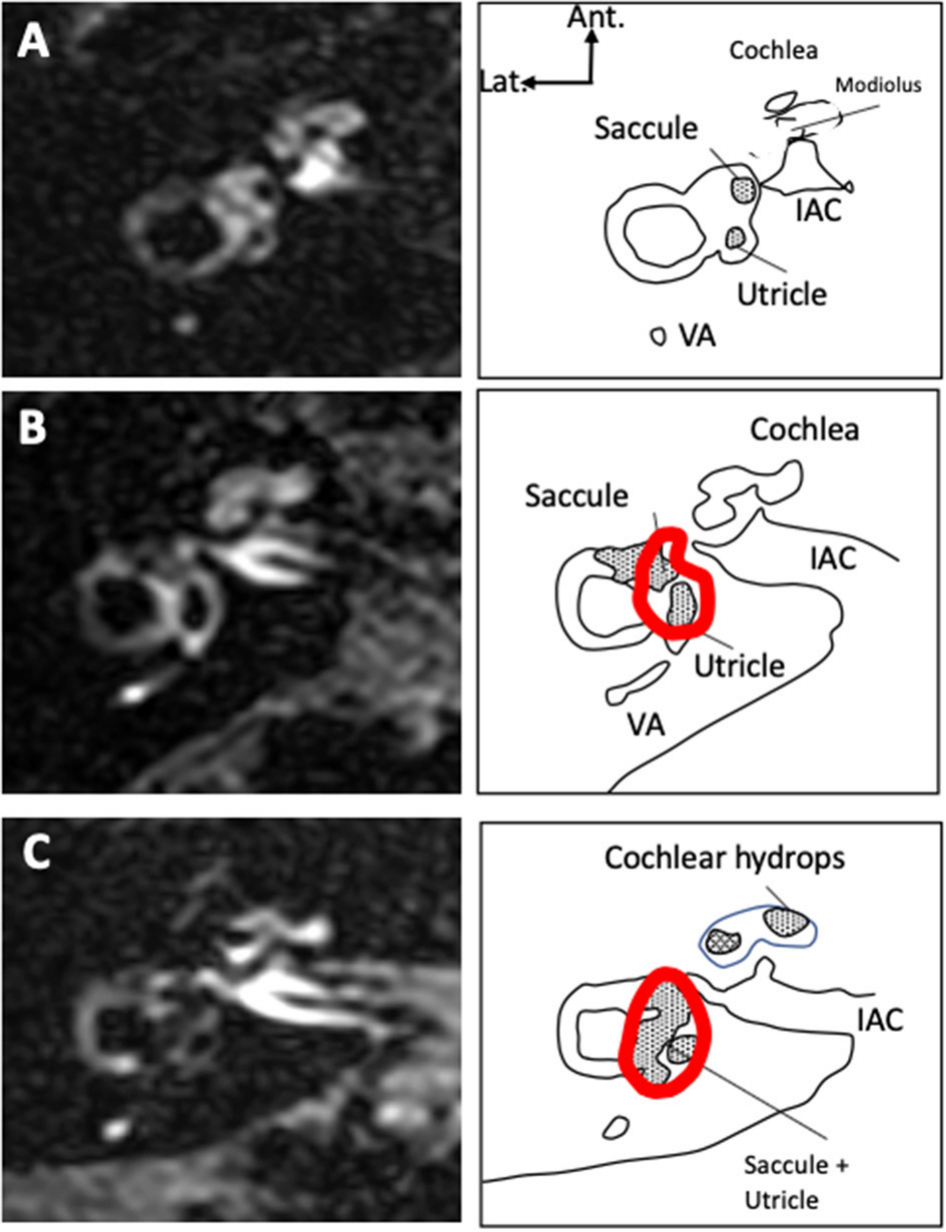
Vestibular and cochlear hydrops on 3D-FLAIR axial views 4 hours after IV gadolinium. Examples of ears with no signs of hydrops [grade 0, (A)], with moderate hydrops [grade I vestibular and cochlear hydrops, (B)], and significant hydrops [grade II vestibular and grade I cochlear hydrops, (C)] are presented. The total surface of the vestibule as measured in the study is contoured in red. Only the saccular surface inside the vestibular area was measured. In case of extensive hydrops (C), the saccule could not be distinguished from the utricle and the surfaces of both structures were measured together.
